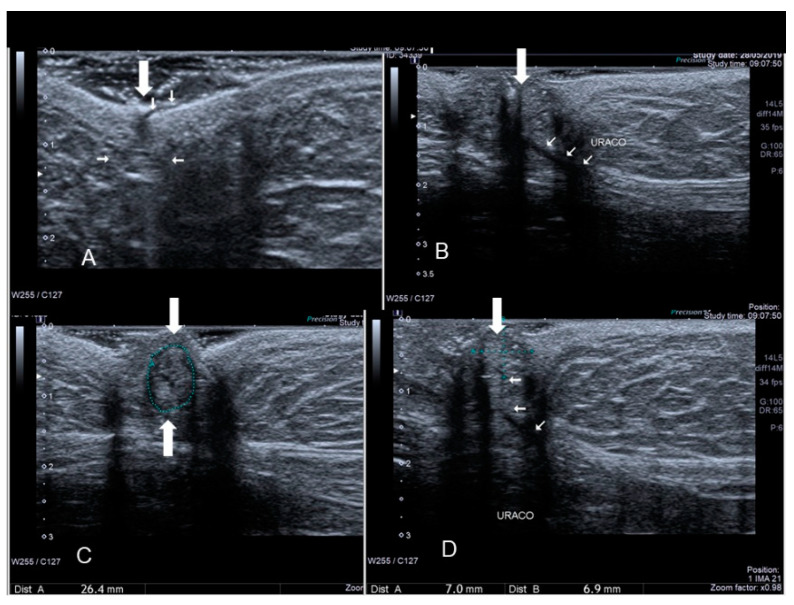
The ultrasonographic appearance of another Villar’s nodule (thick and thin white arrows) (A–D).
